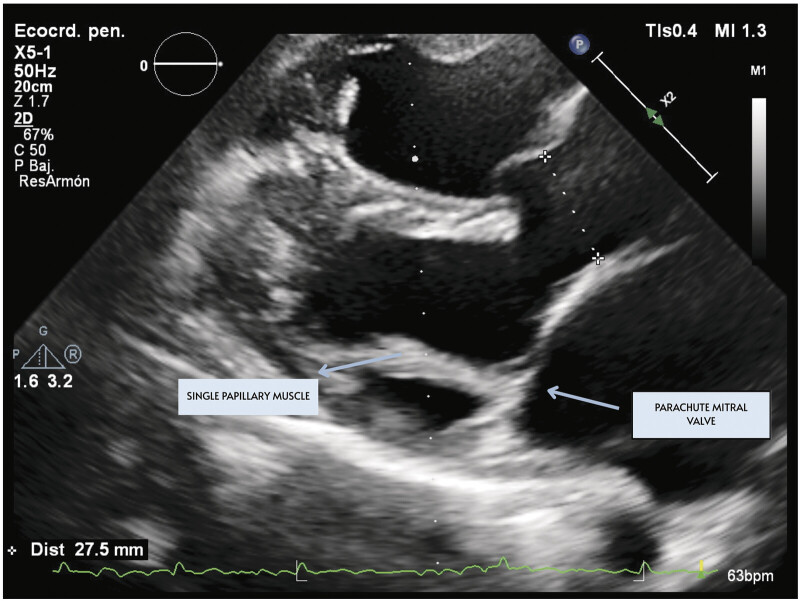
Echocardiographic view showing the mitral valve with chords attached to a single papillary muscle.
